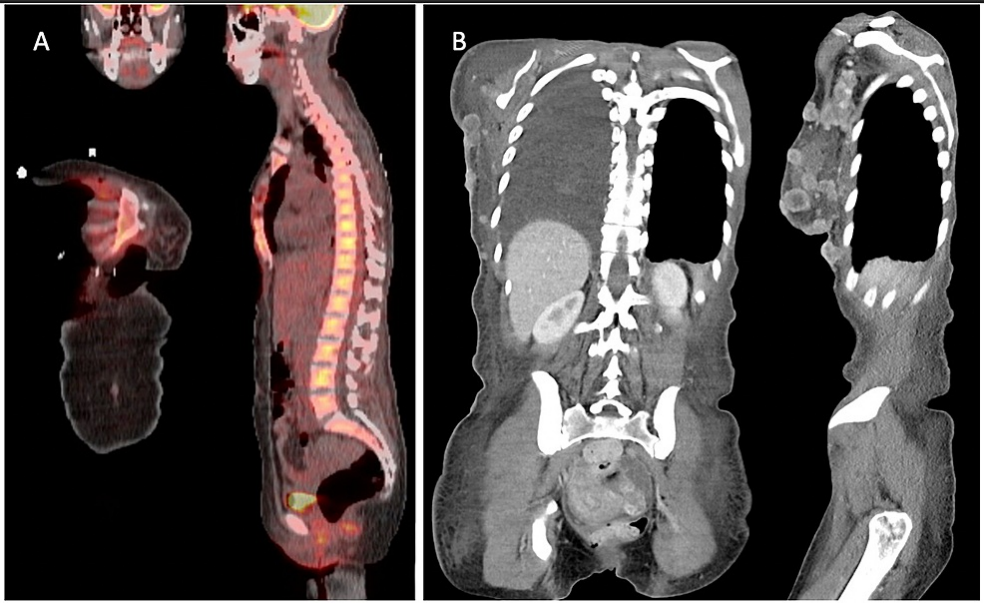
PET imaging on POD1 after initial excision and most recent CT. (A) PET scan after initial excision. Note increased activity along R internal mammary lymph nodes. (B) Most recent CT scan showing extensive subcutaneous disease. PET: positron emission tomography; POD1: postoperative day 1.
